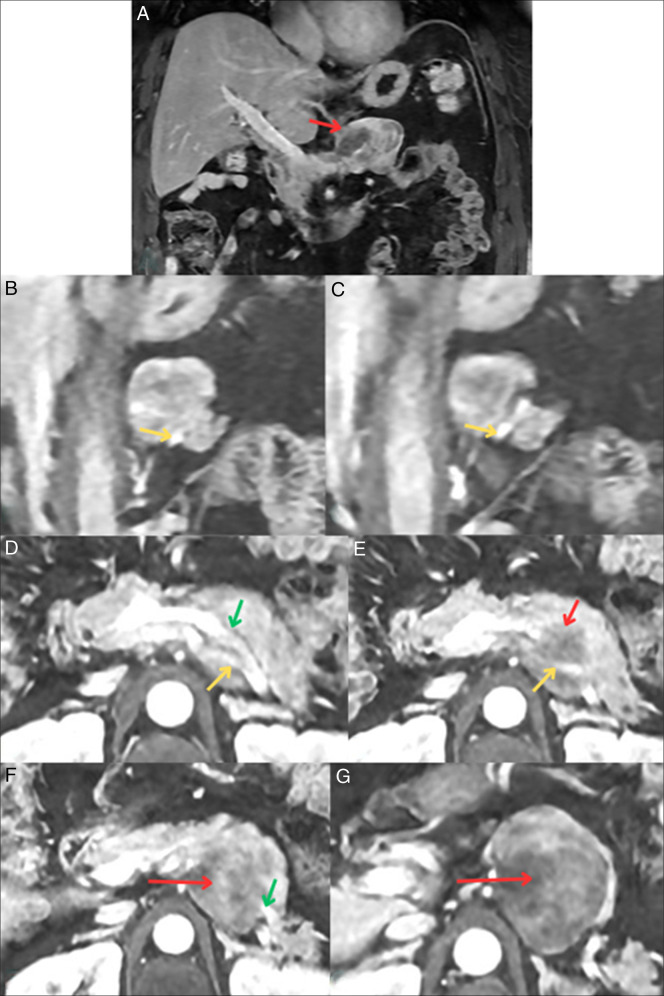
Magnetic resonance imaging abdomen with and without contrast demonstrating coronal views (A–C) of the mass (red arrow) with suggestions toward abutting the splenic vessels: splenic artery (green) and splenic vein (yellow). Axial views (D– G) showing similar findings.
